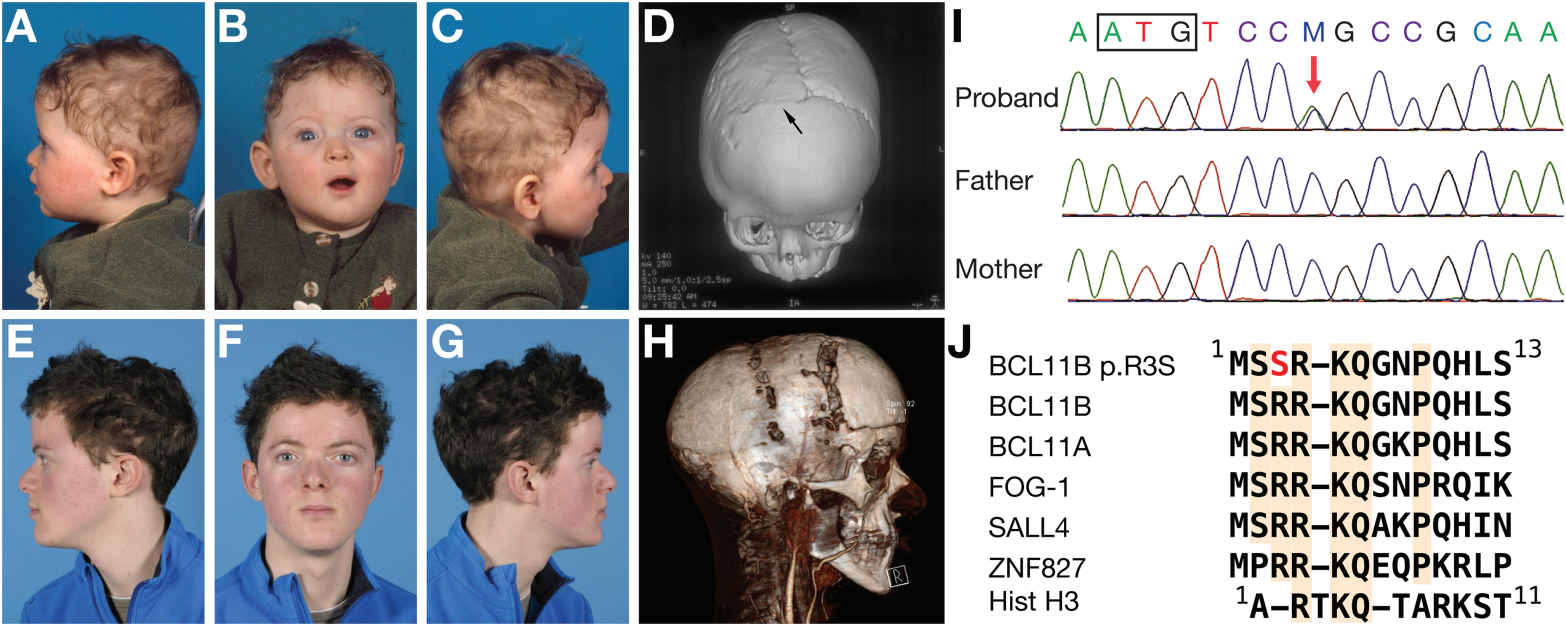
Clinical features. (A–C) Pre-operative facial photographs and 3D-CT scan (D) at the age of 10 months. Right coronal synostosis is indicated by the arrow. (E–G) Post-operative facial photographs at the age of 15 years. (H) 3D-CT and angiogram scan at the age of 12 years just prior to distraction. (I) DNA sequence analysis of proband and parents showing a *de novo* C>A variant (*red arrow*) in *BCL11B* (c.7C>A). The box encloses the start codon. (J) Alignment of amino termini of BCL11B, transcription factors harbouring related sequences and histone H3.
